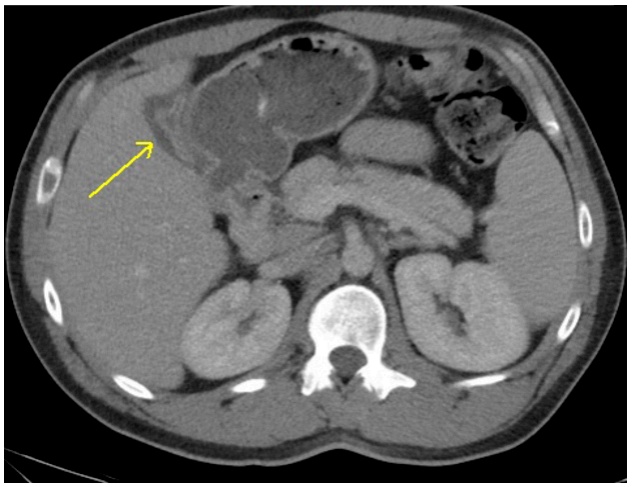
Figure 1 CT Abdomen/Pelvis Axial Slice Demonstrating Gall Bladder Contraction with Wall Thickening
